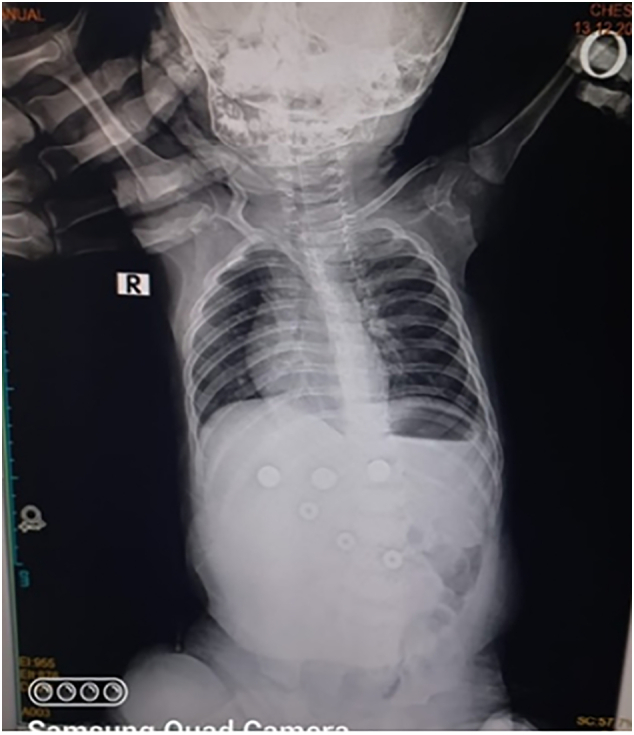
A plain erect abdominal X-ray revealed abnormal air distribution with scanty distal aeration.
